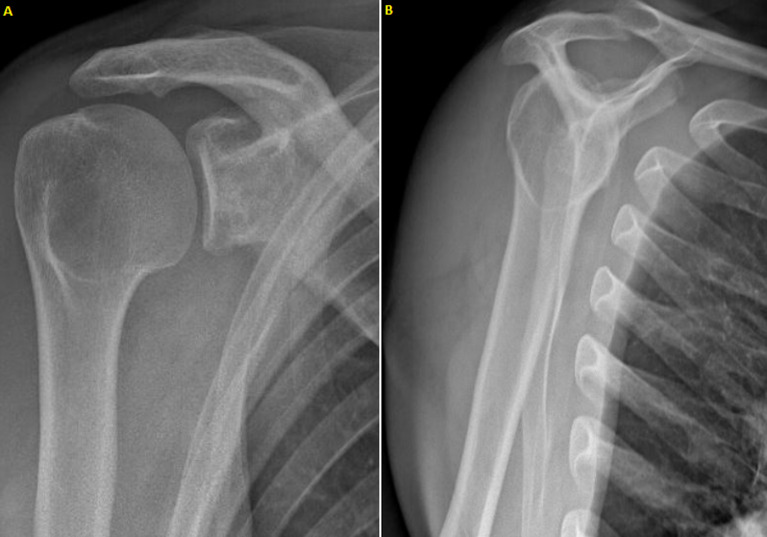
(A,B) X-rays at 12 months post-operatively (lamy side and front)
